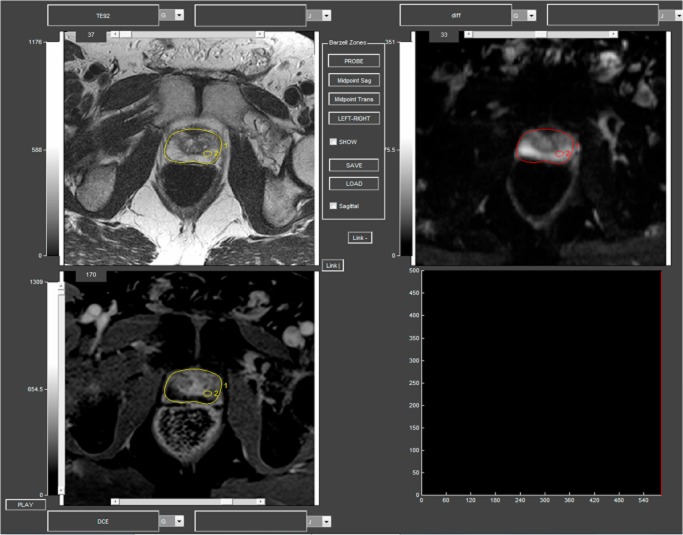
Manual contouring of the MR-visible lesion on axial T2-weighted images from a preoperative mpMRI. Dynamic contrast-enhanced and diffusion-weighted images are also displayed on the same screen. The prostatic capsule (contour 1) (apex to base) and the MR-visible lesion (contour 2) were contoured separately.
